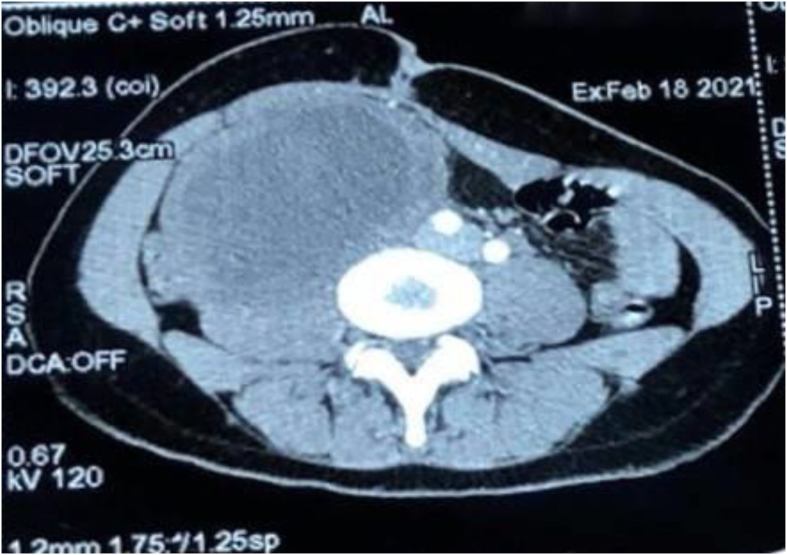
Abdominal CT scan showing the retroperitoneal Schwannoma.
